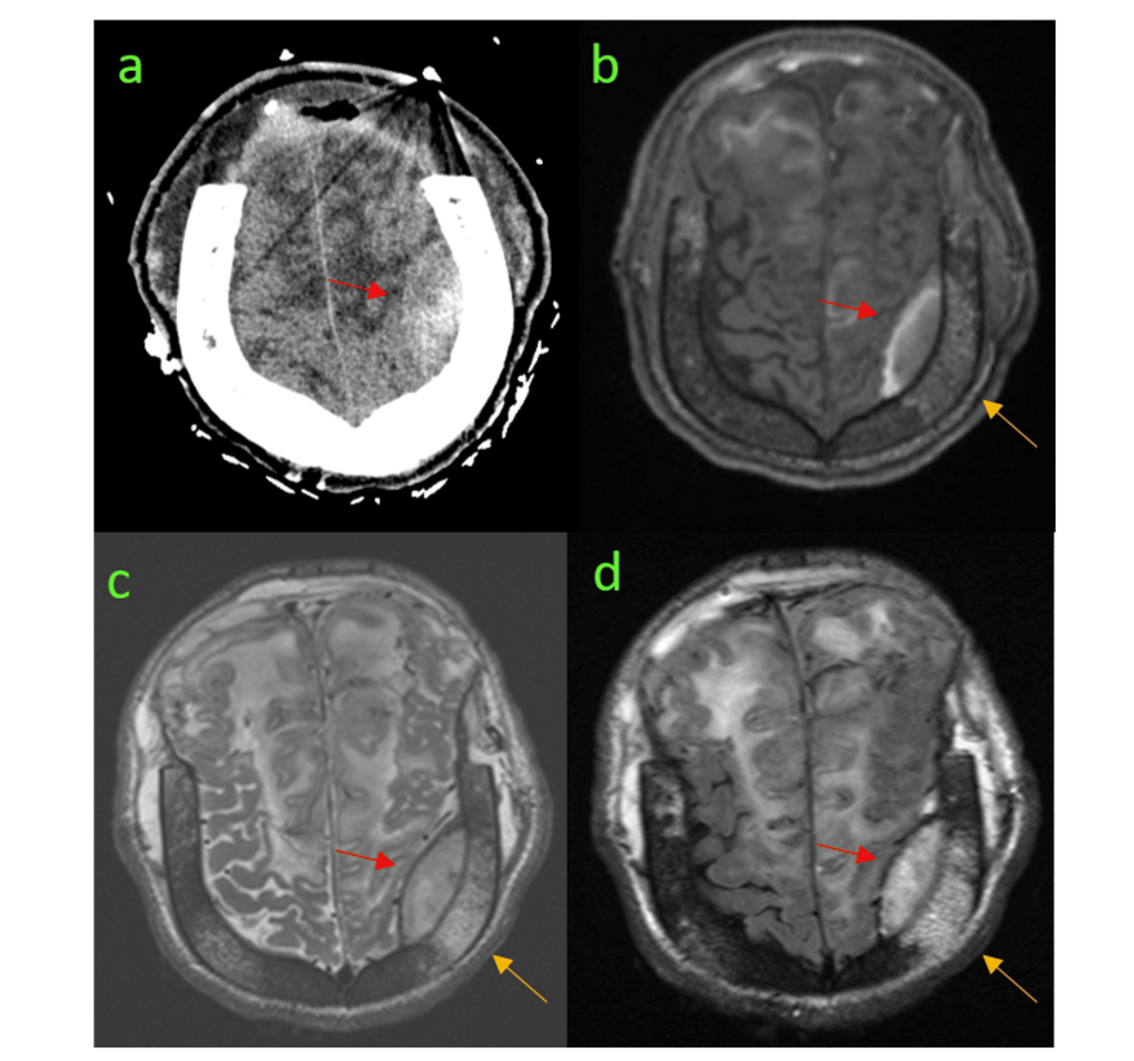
Bone Necrosis Over Epidural Hematoma (a) Post-operative CT scan showing epidural hematoma (EDH) (red arrow). (b-d) MRI images showing bone infarct (yellow arrow) above parietal EDH (red arrow) in T1 (b), T2 (c), and FLAIR (d). FLAIR: fluid-attenuated inversion recovery
